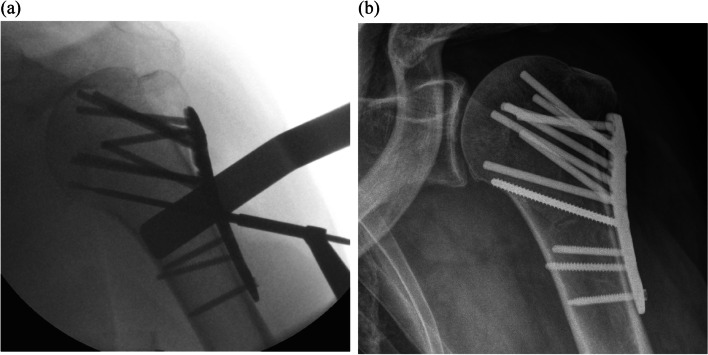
Intra-operative (a) and post-operative (b) radiographs of variable angle calcar screw positioning in the inferior cortex with the ALPS plating system
